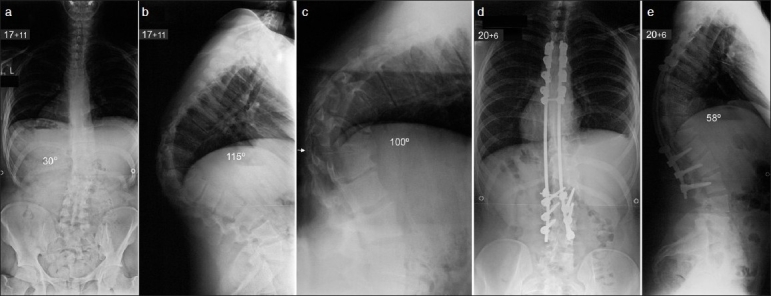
Preoperative posteroanterior radiograph (a) of Patient 1 shows a mild right thoracolumbar scoliosis measuring 30°. Lateral radiograph (b) shows a severe thoracolumbar kyphosis measuring 115°. Preoperative supine lateral radiograph in hyperextension against the bolster (white arrow, (c) shows the kyphosis correct to only 100°. Posteroanterior (d) and lateral (e) radiographs at 2.6-year-follow-up show normal coronal balance of the spine and a residual kyphosis of 58°
